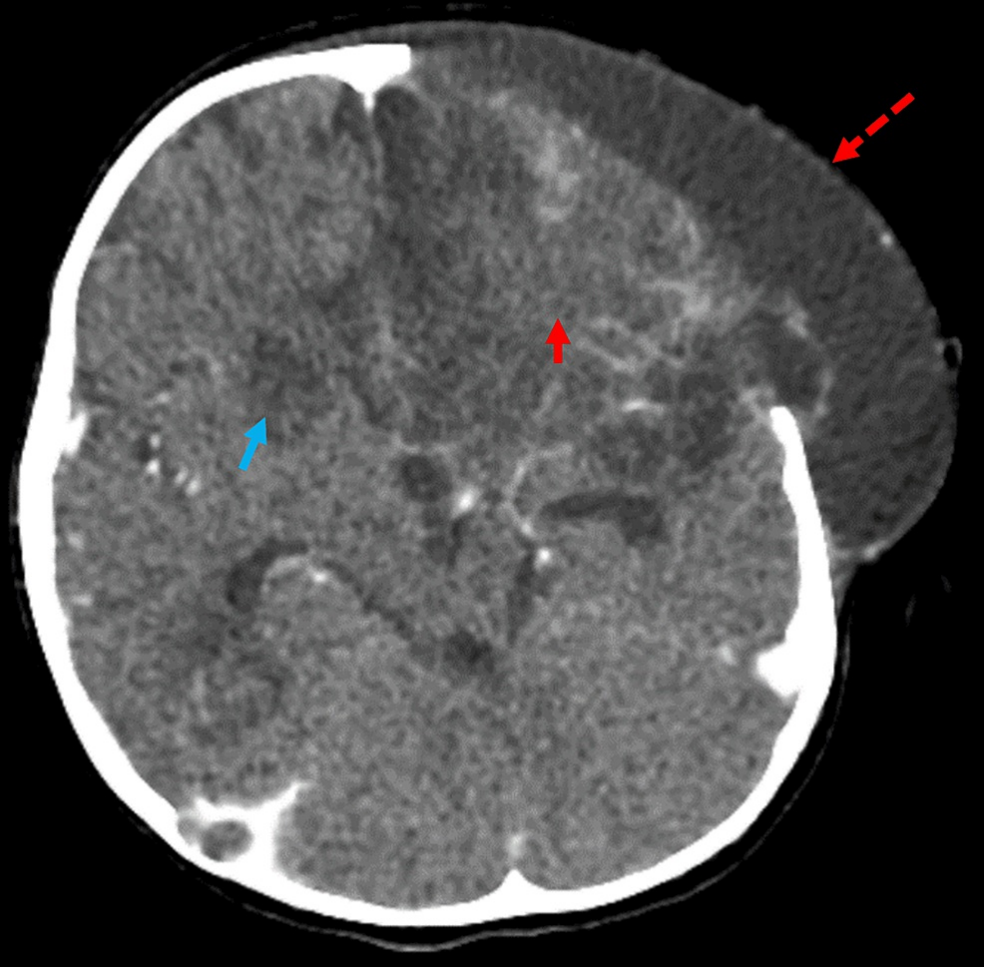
CT of the brain Subgaleal CSF attenuation suggesting hygroma formation (dashed arrow). Left frontoparietal encephalomalacia changes (red arrow). Right frontal encephalomalacia changes (blue arrow). CT: computed tomography, CSF: cerebrospinal fluid
